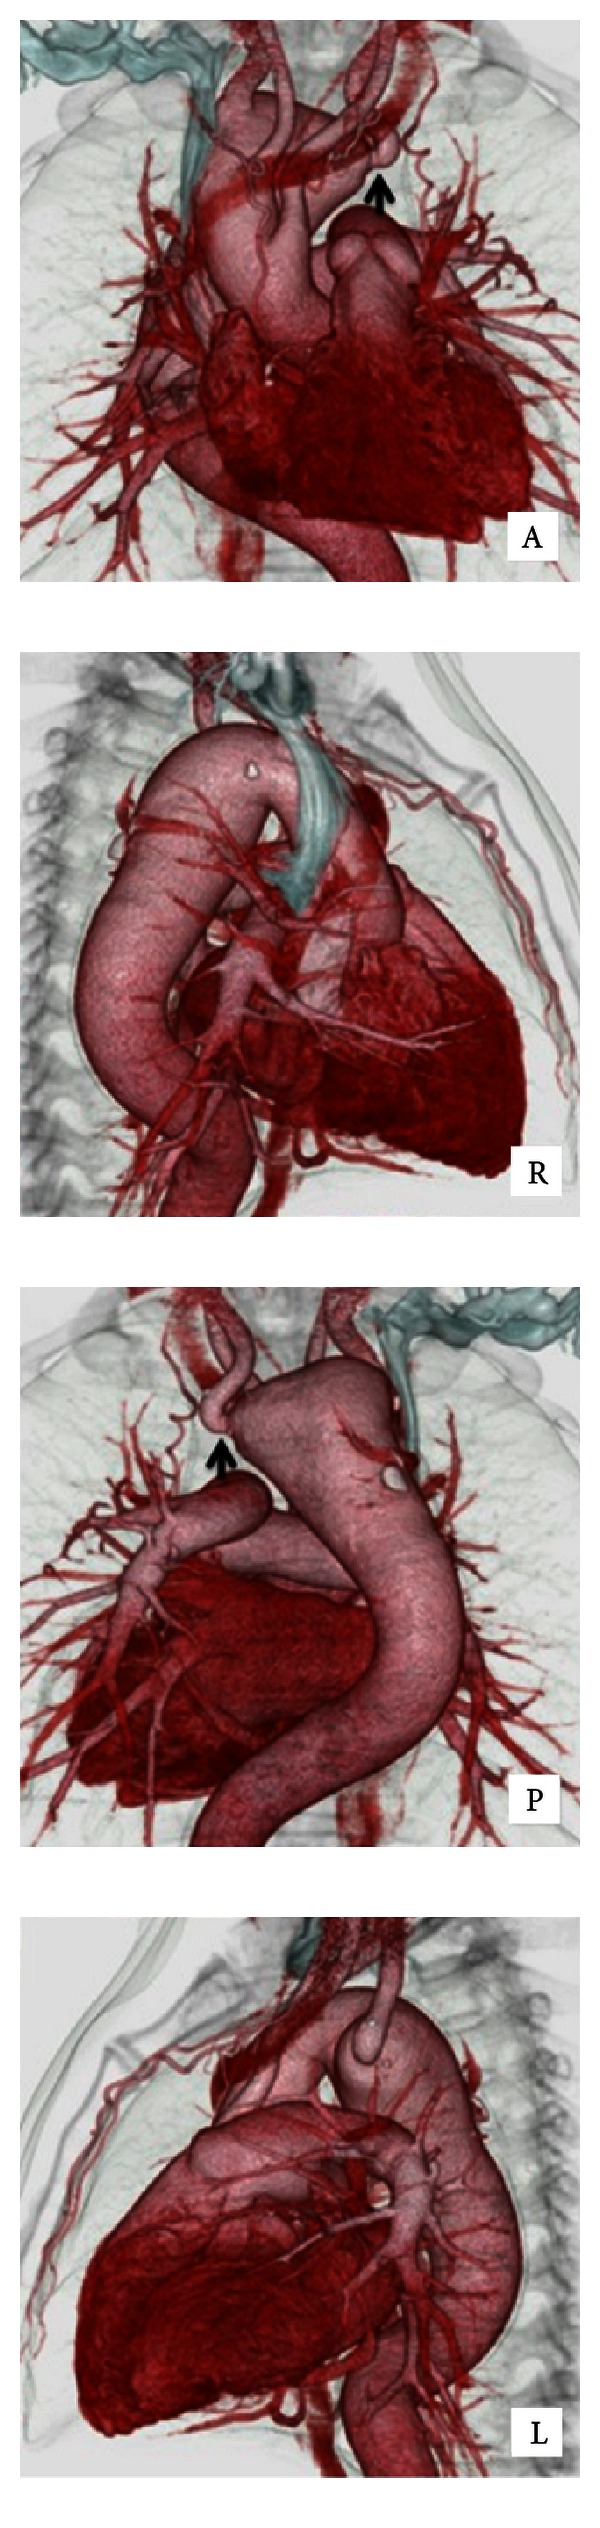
Enhanced CT showed right aortic arch, Kommerell diverticulum, and aberrant left subclavian artery. There was a 50% stenosis of the orifice of aberrant left subclavian artery (arrows).
